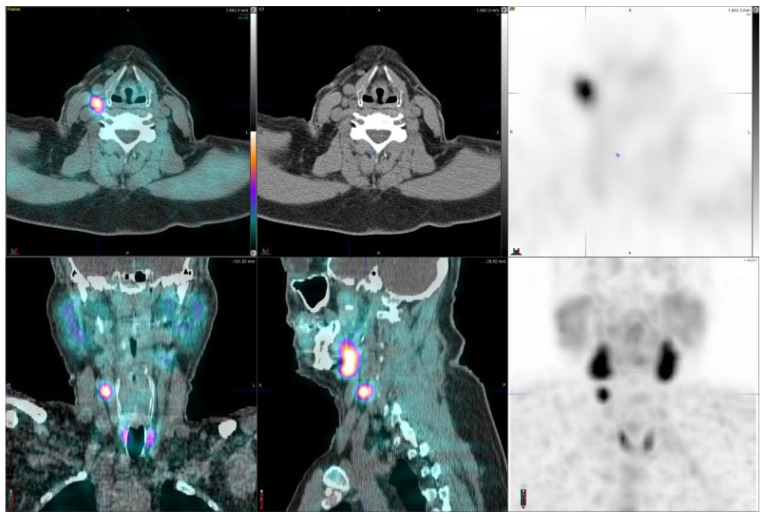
Parathyroid adenoma, in the right lateral mid neck, weighing 1.54 g. This 53-year-old male had S-PTH concentration of 125 ng/L and S-Ca of 11.0 µg/L. The SUVmax values of the right-sided adenoma were 7.8 (CGZAS) and 4.7 (CGAS), respectively. Visually, this uptake was high (TBR > 3) and lesion diameters on CT were 9 mm × 21 mm.
